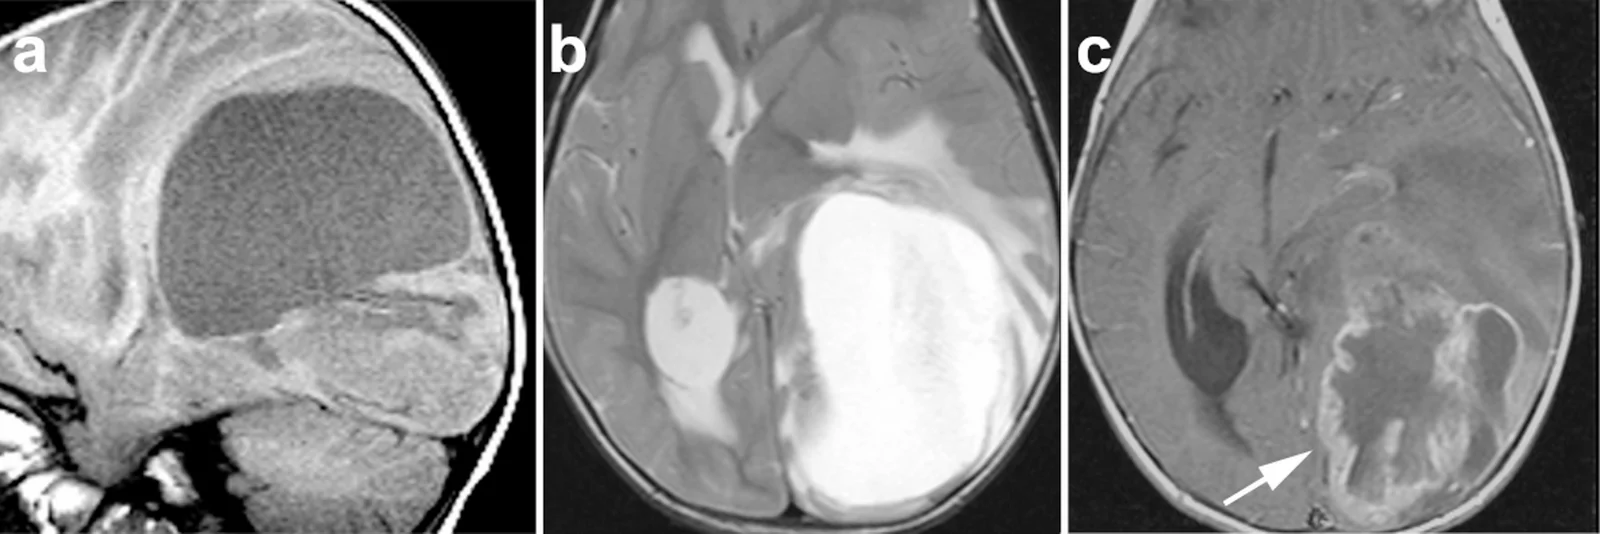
A case of supratentorial ependymoma. Sagittal T1-weighted image (a), axial T2-weighted image (b), and contrast-enhanced axial T1-weighted image (c). A large cystic lesion is seen in the left occipital lobe with peripheral enhancement (arrow). Peritumoral edema is present, accompanied by a midline shift to the right
